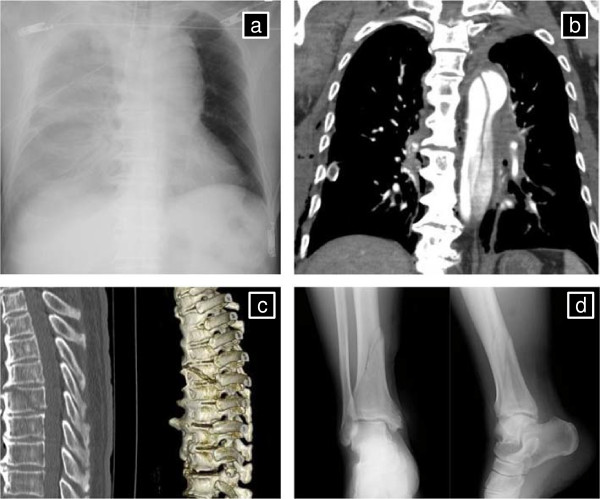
Details of injuries. (a) Chest X-ray showing massive right hemothorax. (b) CT reconstruction showing traumatic aortic dissection. (c) CT reconstruction showing fracture of the eighth and ninth thoracic vertebrae. (d) X-ray showing fracture of the right tibia.
